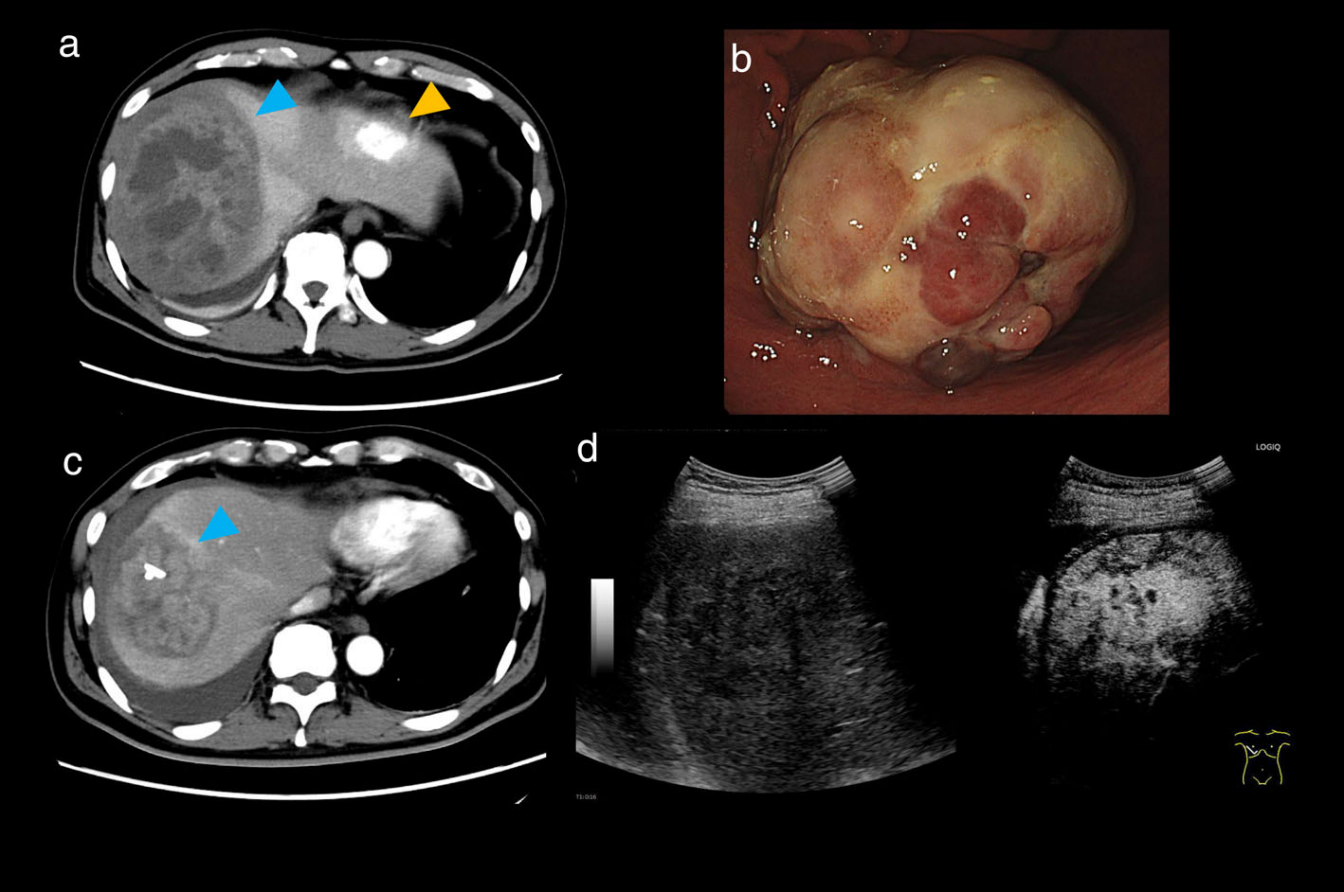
Abdominal contrast‐enhanced computed tomography (CECT) shows a 10‐cm mass with encapsulated fluid in the right hepatic lobe below the diaphragm (a, blue arrowhead) and a hemangioma in the left hepatic lobe (a, yellow arrowhead). Upper gastrointestinal endoscopy imaging shows a contrast‐enhanced 6‐cm‐large mass within the gastric body (b). CECT 2 weeks after drainage shows strong enhancement of the thick septum (c, blue arrowhead). Contrast‐enhanced ultrasonography revealed strong intratumoral enhancement in the arterial phase (d).
